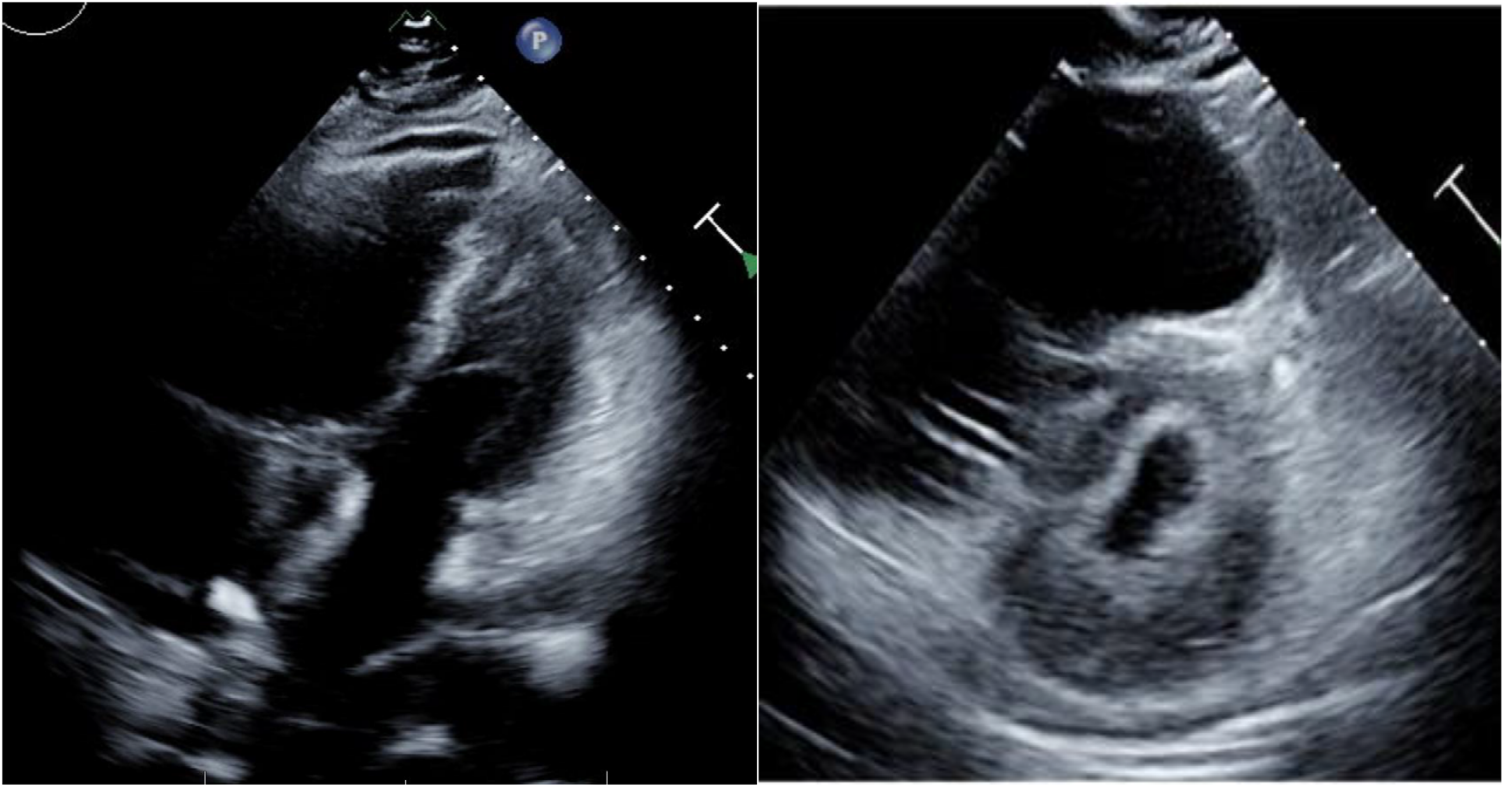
Four‐chamber apical and parasternal short views demonstrating severe dilation of right atrium and right ventricle (RV)
